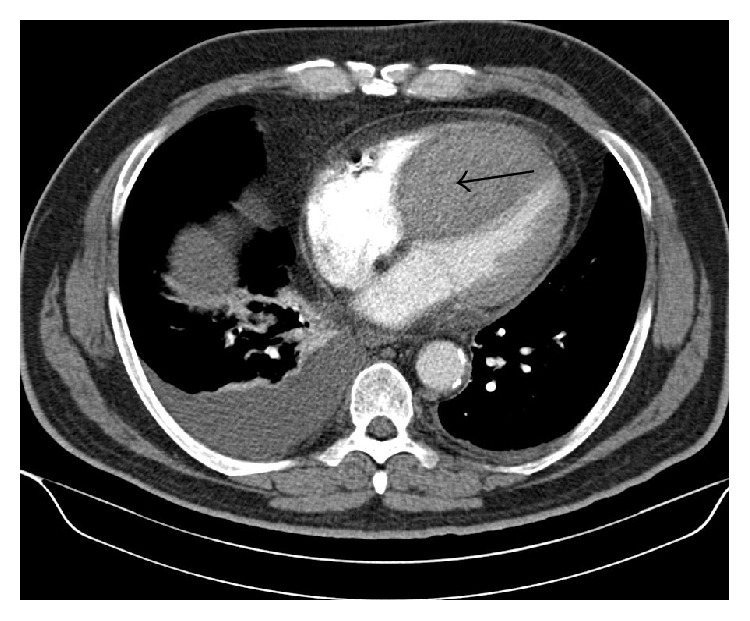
CT scan confirms a huge intraventricular septum-hematoma.
